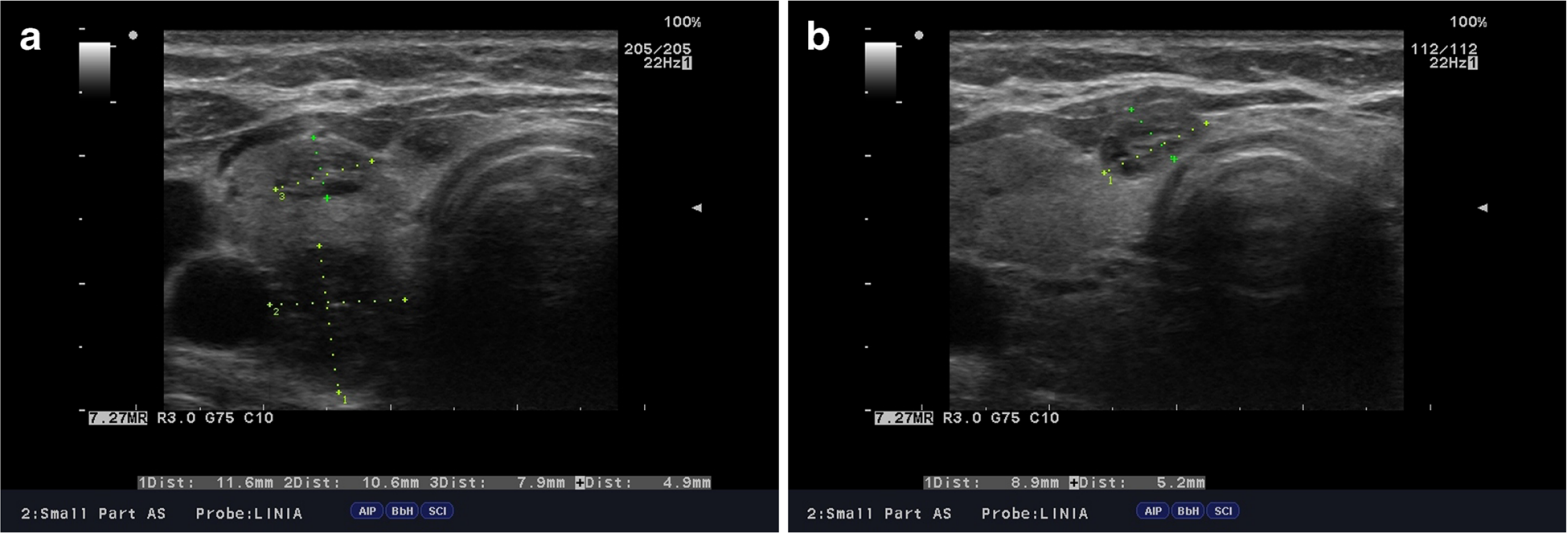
(a,b) Ultrasound image of right thyroid lobe with the features of nodular remodeling in 57-year-old female with SLL-B/CLL-B.
